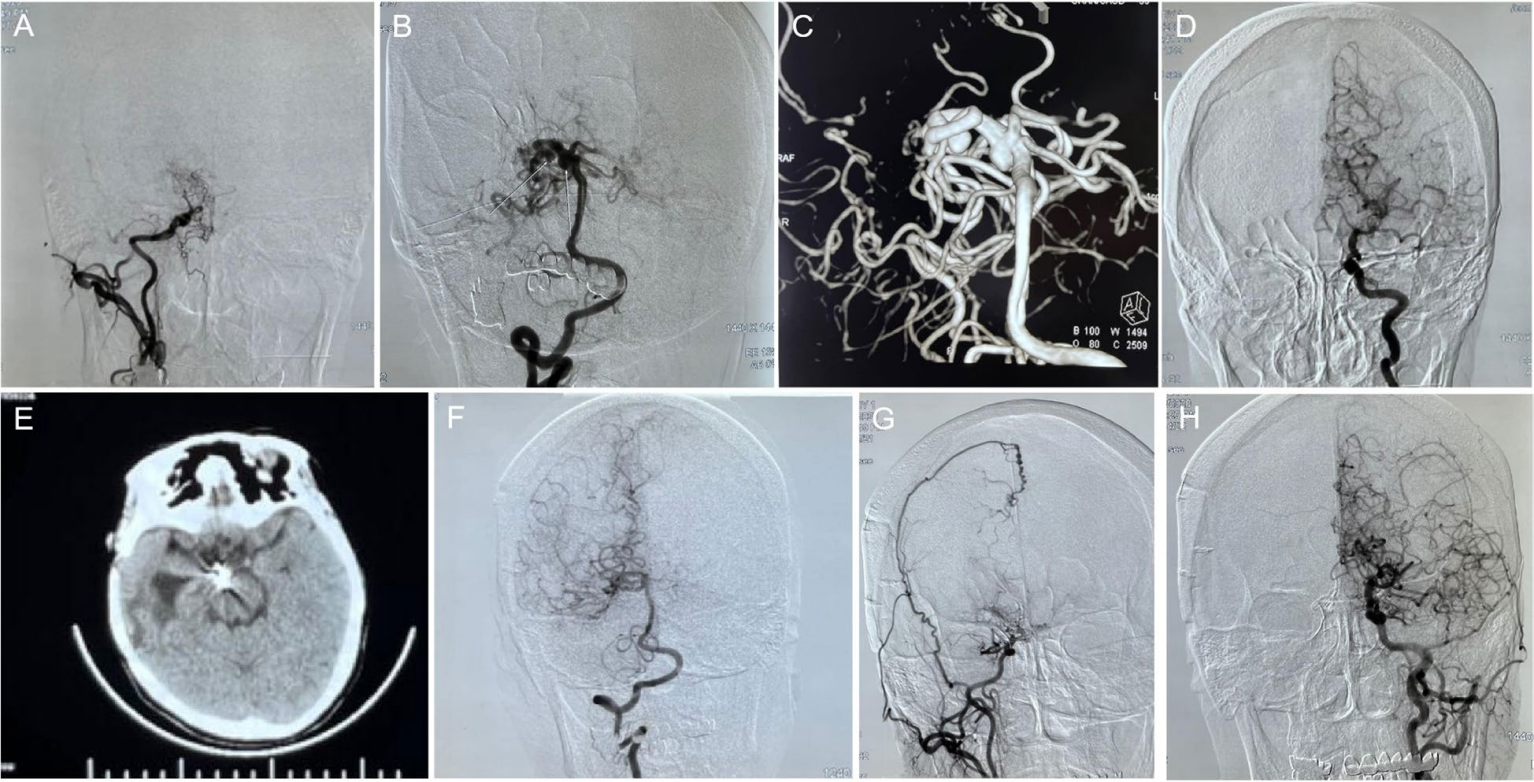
Typical magnetic resonance imaging for Moyamoya disease (MMD)-related intracranial aneurysm (IA). A–D figures referred to the preoperative neuro-image, and E–H figures referred to the postoperative neuroimage. A Digital subtraction angiography (DSA) test for the right common carotid artery (CCA) showed the MMD lesion. B DSA test for the right vertebral artery (VA) showed 2 aneurysms. C 3D-DSA for the VA. D DSA for the left internal carotid artery (ICA). E Postoperative computer tomography (CT) scan. F Postoperative DSA test for the right (VA). G Postoperative DSA test for the right CCA. H Postoperative DSA test for the left ICA
